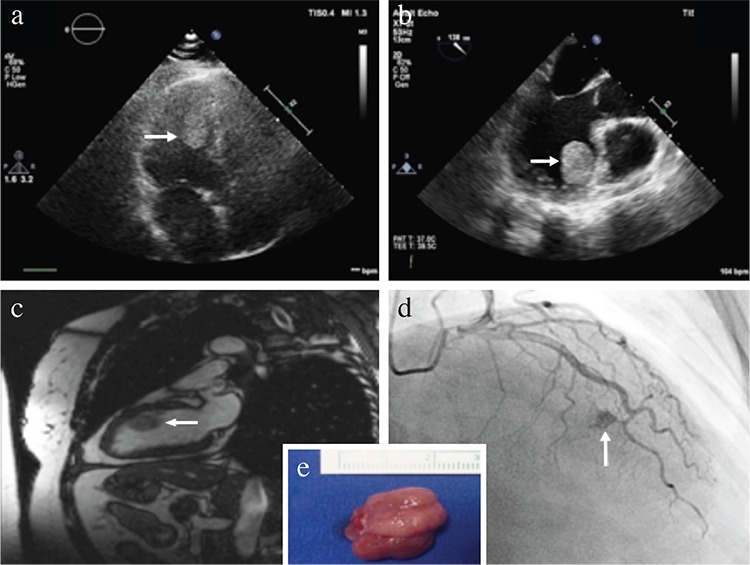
Preoperative transthoracic echocardiography; apical four-chamber view showing a hyperechoic, round, mobile mass (arrow) (a). Transesophageal echocardiography revealed a homogenous, round, pedunculated mass (arrow) originating in the anteroseptal wall of the left ventricle (b). A round, hyperintense mass (arrow) originating in the anteroseptal wall of the left ventricle is seen on the two-chamber white blood cardiac magnetic resonance imaging (c). Preoperative coronary angiography demonstrated the characteristic finding of cardiac hemangioma “tumor blush” (arrow), which highlights the vascular nature of the tumor (d). Image of the excised cardiac mass measuring 2.3×1.5×1.0 cm (e).
